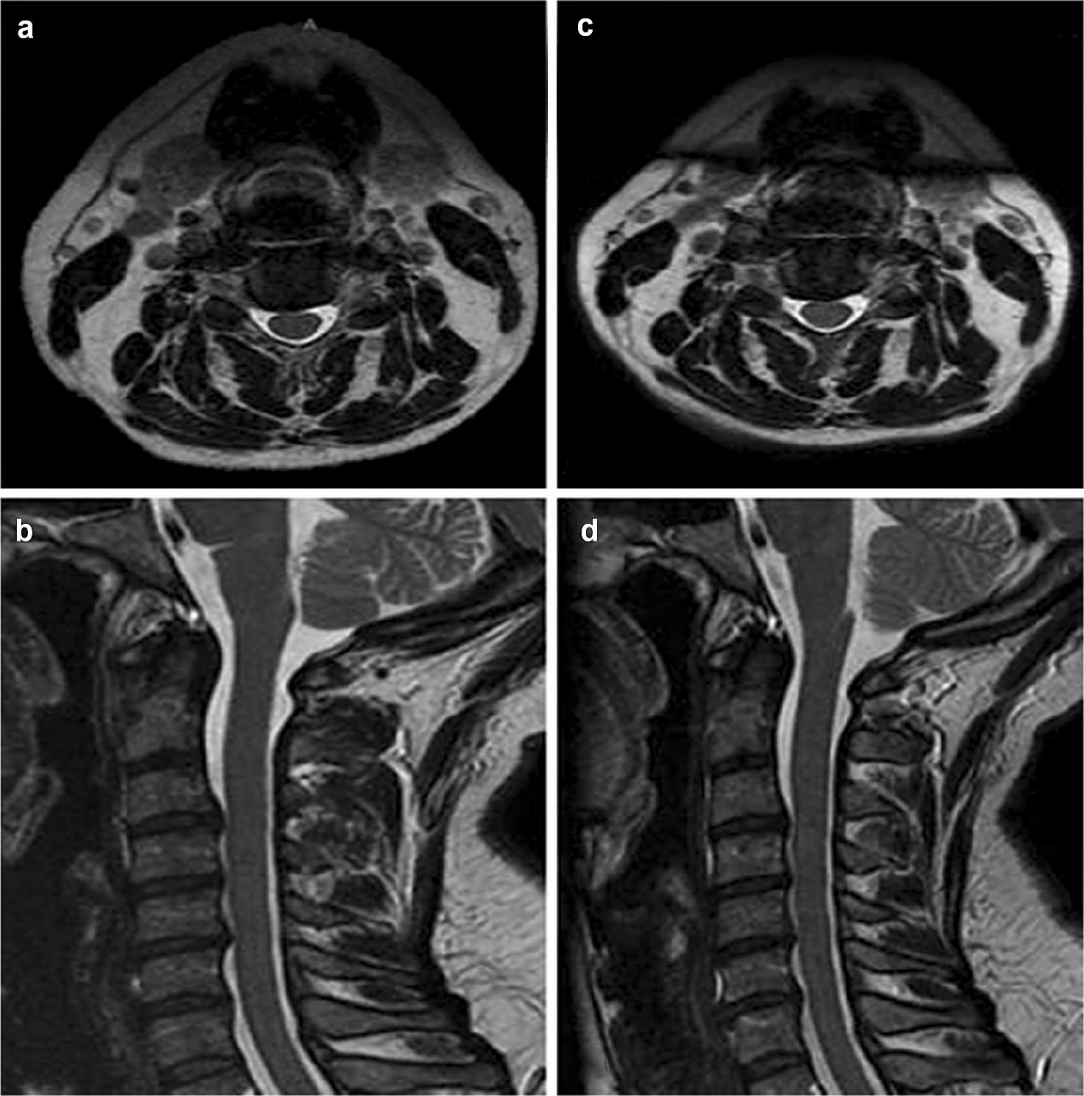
Axial and sagittal T2 MR images pre-treatment (a, b) and post-treatment (c, d) in a 46 female patient with pain reduction more than 50%: in this case, we had not significant MRI changes of the discal protrusion, despite of clinical improvement
